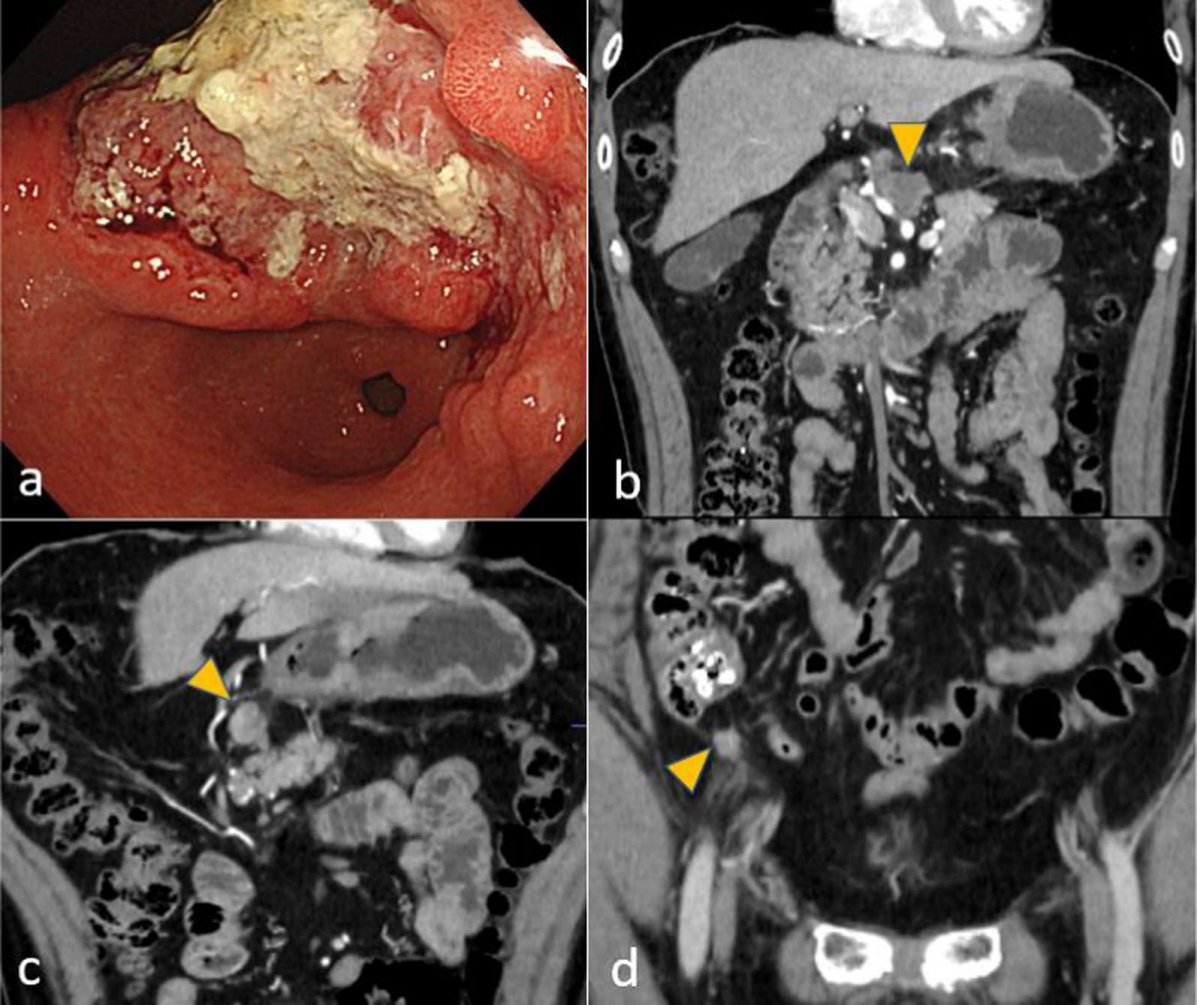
Findings of case 2 with gastrointestinal endoscopy and abdominal computed tomography before treatment. a A type 3 tumor in the lesser curvature of the upper to lower gastric body was found. b–d Enlarged station No. 6 and 8a lymph nodes and peritoneal metastasis near the cecum (arrowhead) were found
